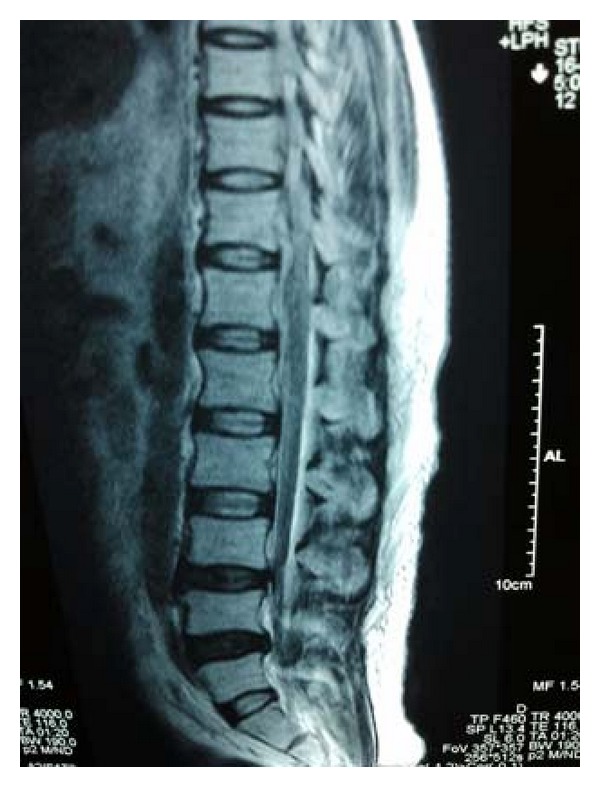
MR imaging of dorsolumbar spine did not reveal any abnormalities, with no evidence of compressive myelopathy.
